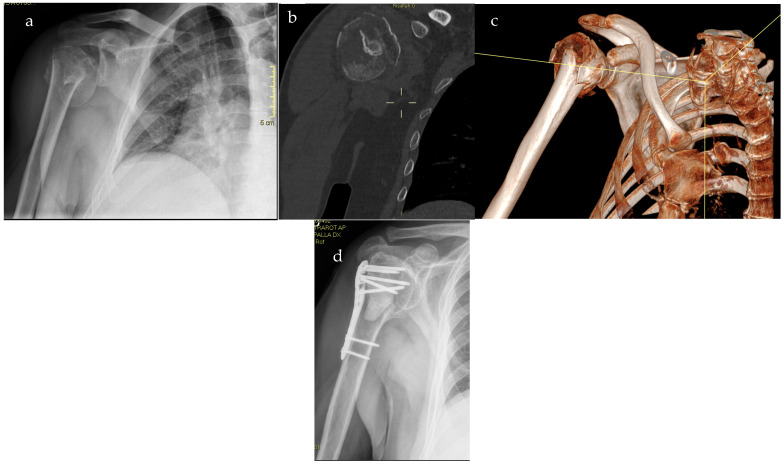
(a) Radiographic images; CT scan (b) and 3D CT (c) reconstruction of fracture with varus breakdown and bone gap. (d) Post-operative evaluation; the presence of the device replaces the loss of substance.
